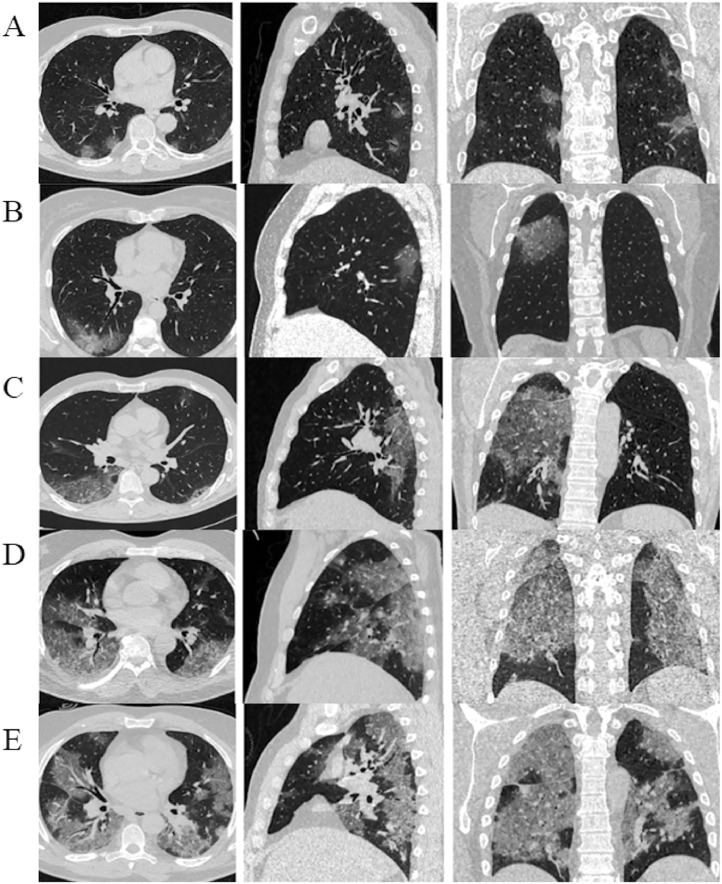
Chest computed tomography images of patients with COVID-19 with varying degrees of lung involvement. A: <10% of involvement (one point), B: 10-25% of involvement (two points), C: 26-49% of involvement (three points), D: 50-75% of involvement (four points), and E: >75% of involvement (five points), respectively.
